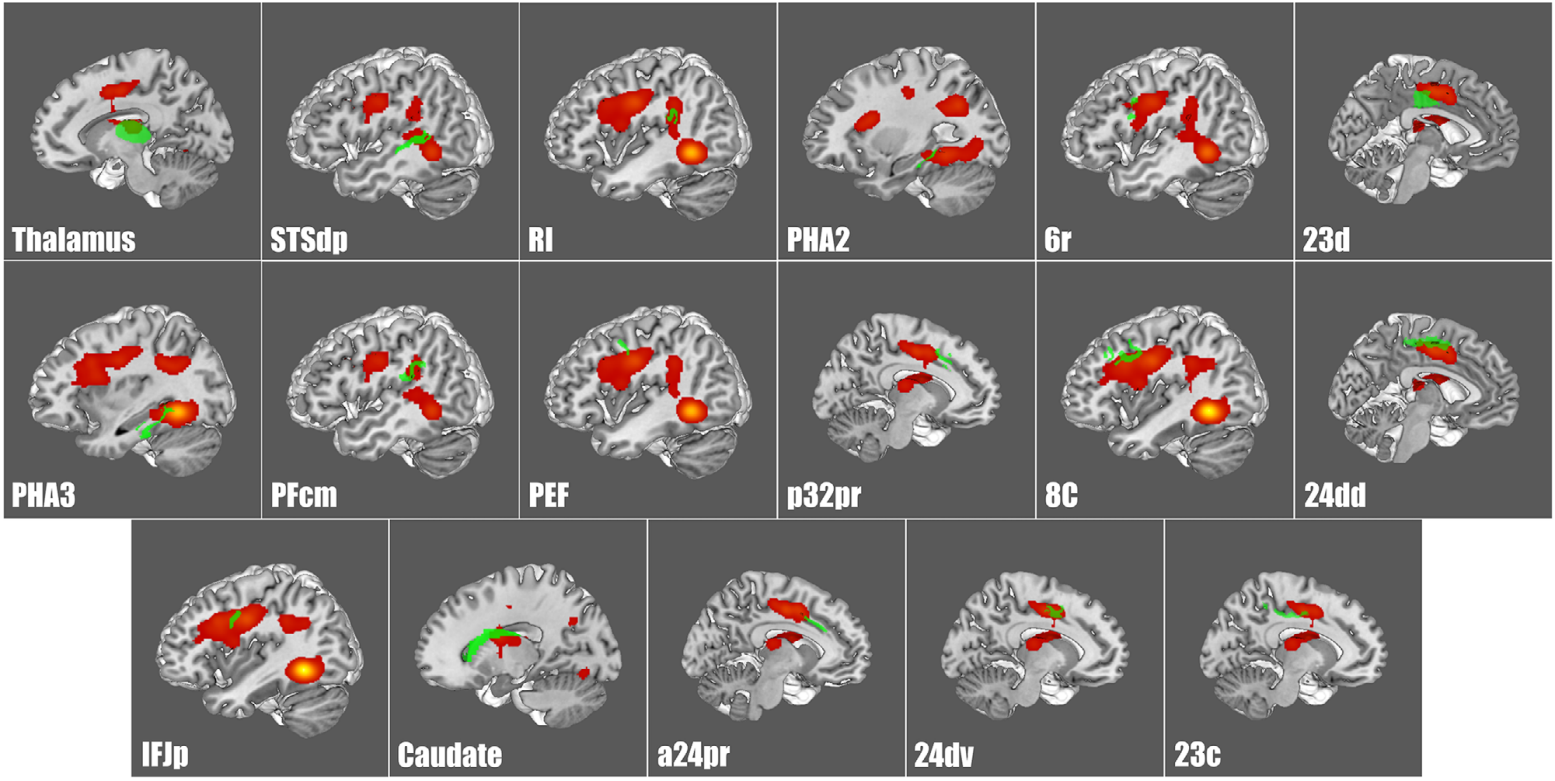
Activation likelihood estimation of task‐based functional MRI experiments related to area PH. The three‐dimensional ALE data (red) are displayed in Mango on a brain normalized to the Montreal Neuroimaging Institute coordinate space, with overlapping Human Connectome Project parcellations (green). Here, ALE data represent areas that coactivate with PH. ALE, activation likelihood estimation; 8c, Brodmann area 8 component C; PEF, prefrontal eye field; IFJp, inferior frontal junction area/posterior; 6r, rostral premotor area 6; p32pr, posterior 32 prime; a24pr, anterior 24 prime; 24dv, ventral area 24d; 24dd, dorsal area 24d; 23c, Brodmann area 23 component C; 23d, Brodmann area 23 component D; PFcm, Brodmann area 40 PFcm complex; RI, retroinsular; STSdp, superior temporal sulcus dorsal posterior; PHA2, parahippocampal area 2; PHA3, parahippocampal area 3.
